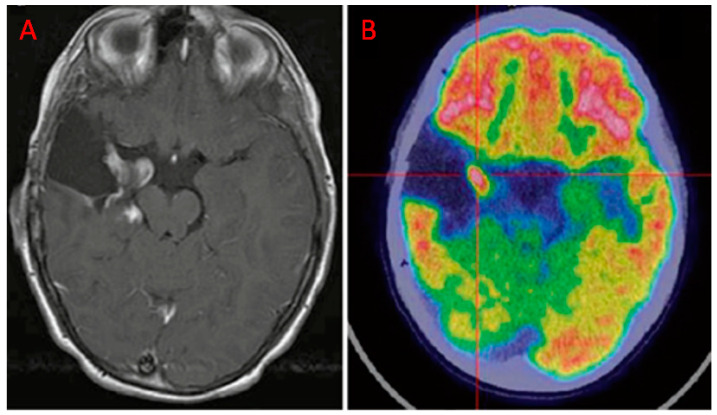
Patient with anaplastic astrocytoma, resected 2 years before, treated with temozolomide. (A) T1-w MRI signal alteration in the right amygdala and posterior part of the para-hippocampus. (B) ^18^F-FDG-avid finding, suggestive for disease recurrence (Courtesy of Dr. Cistaro).
